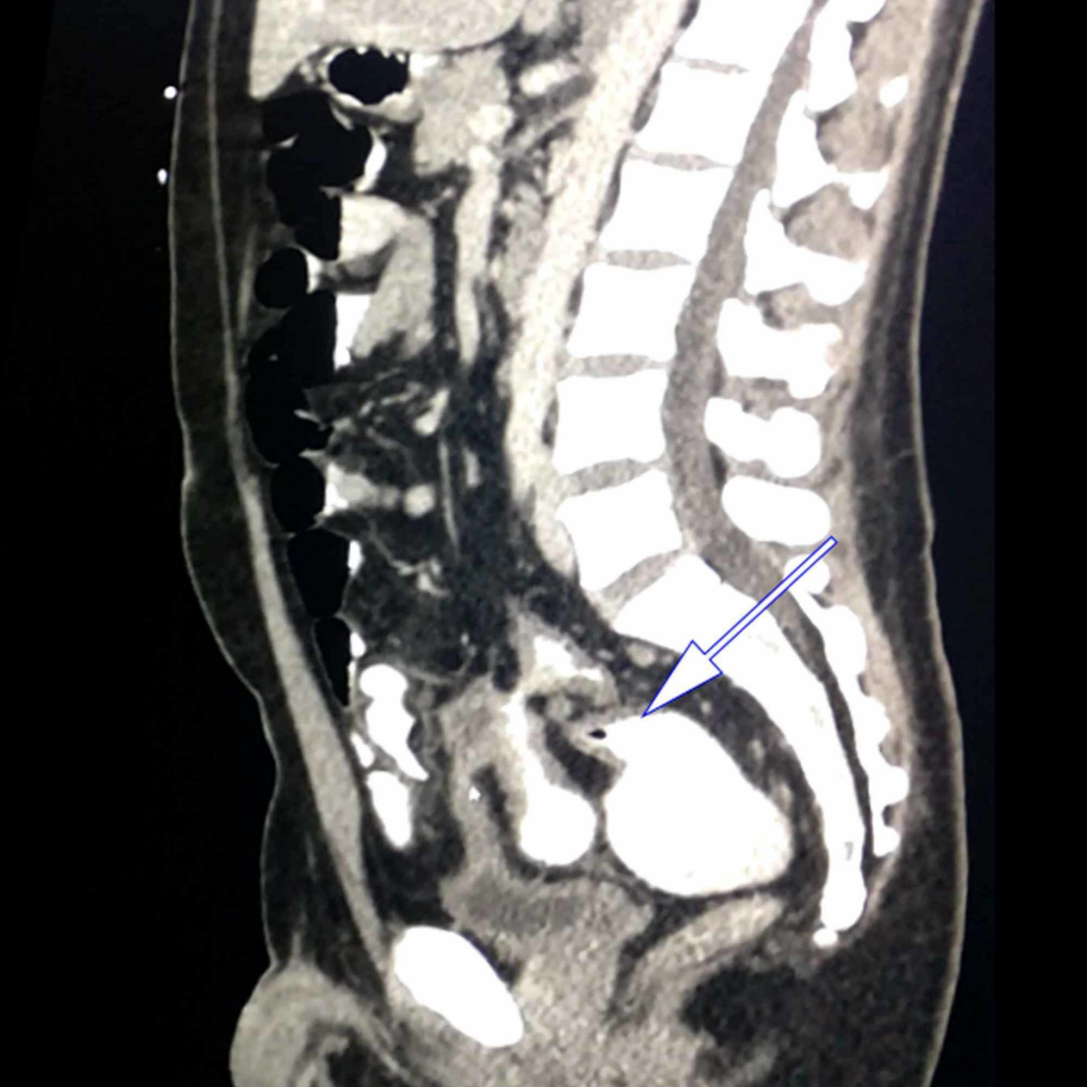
CT scan showing thickened sigmoid colon indicated by the white arrow
